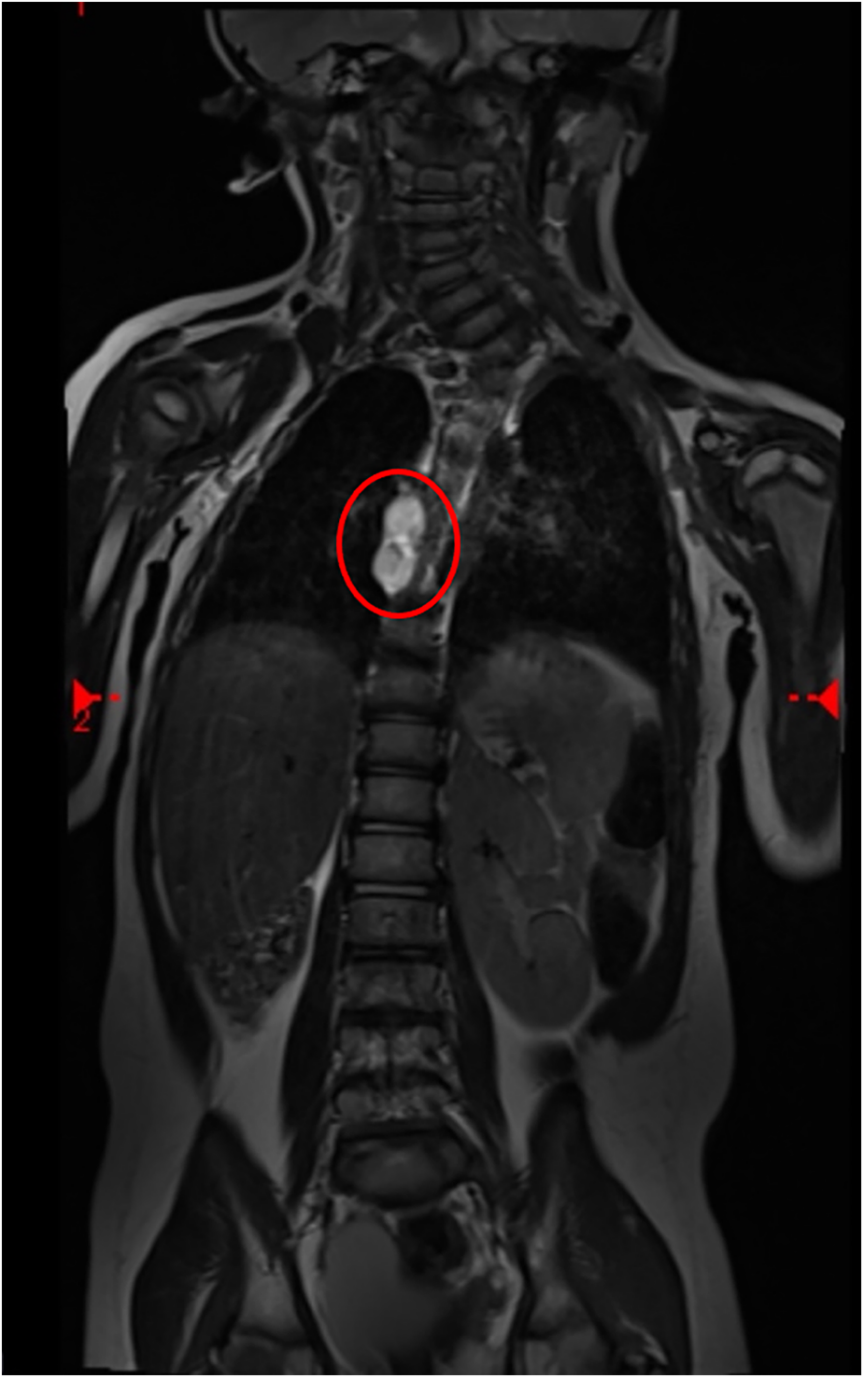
MRI scan of cystic lesions of the esophagus (circle: EDC at the D7–D9 level).
